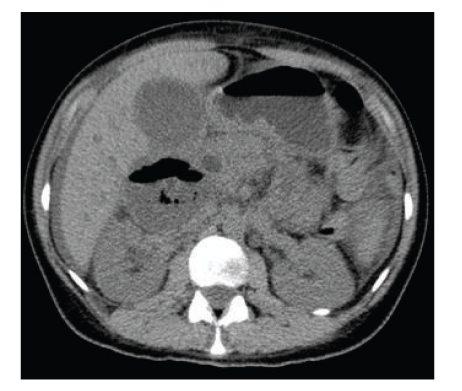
CT scan slice showing the air-fluid filled cyst.
